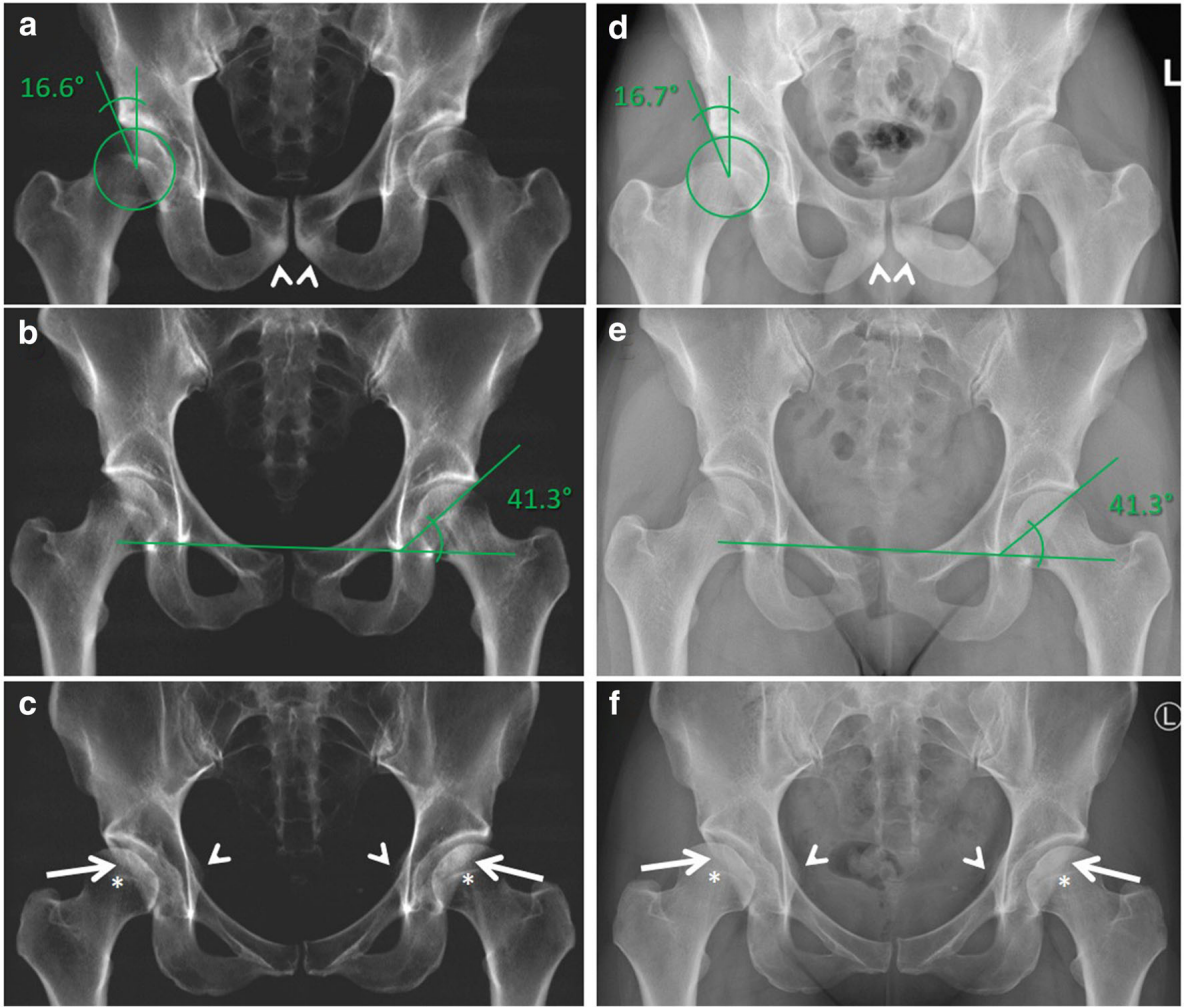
Calculated virtual a.p. pelvic radiographs of the ultra-low-dose CT (a–c) and corresponding digital a.p. radiographs of the pelvis (d-f) in 3 different patients. A 28-year-old male (a and d) with hip dysplasia on both sides. Measurement of the right center-edge angle was almost identical with 16.6° for the virtual (a) and 16.7° for the digital radiograph (d). Note the sacrum and the sclerosis of the inferior pubic bones adjacent to the pubic symphysis (arrowheads) is better visible on the VR, because of bowel gas and soft tissue overlay on the DR. A 24-year-old female (b and e) with normal configuration of the left hip. The Sharp angle was within the normal range and was measured identical with 41.3° on VR (b) and DR (e). A 30-year-old female (c and f) with acetabular retroversion on both sides. VR (c) and DR (f) both show a crossover sign (arrows), posterior wall sign (the posterior acetabular wall is located medial to the center of the femoral head (asterisks)), and ischial spine sign (arrowheads) on both sides. DR, digital radiograph; VR, virtual radiograph
